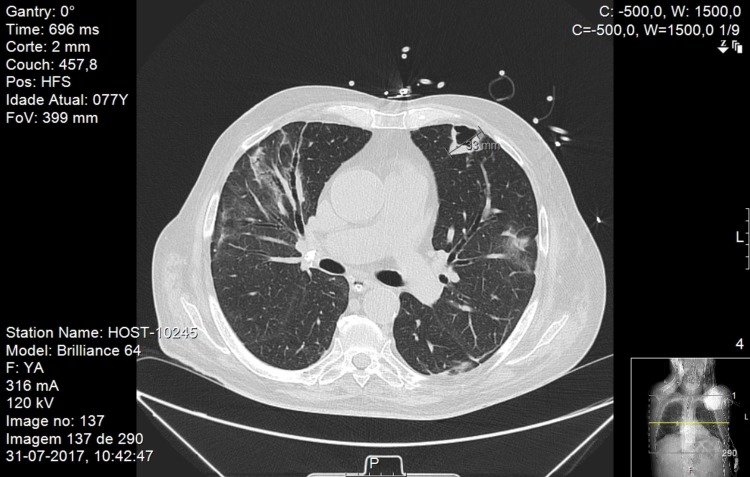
Thoracic CT scan Thoracic computerized tomography scan with cavitation with 3,3 x 3,2 cm on the anterior area of the left upper lobe
